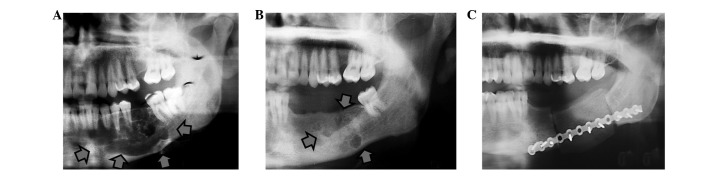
(A) Panoramic radiograph showing a multicystic ameloblatoma in the left mandibular body (arrows). (B) Recurrent ameloblastoma at three sites in the mandibular body (arrows). (C) Radiograph after segmental mandibulectomy and bone reconstruction with iliac crest graft.
